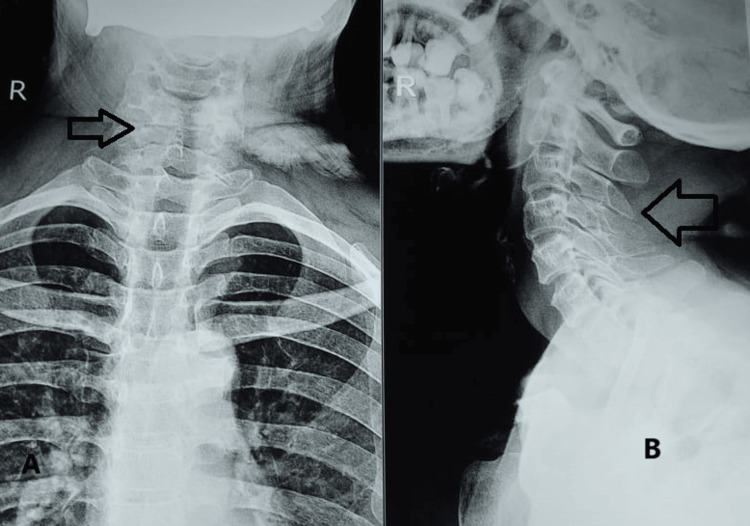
X-ray of the cervical spine in the anteroposterior (A) and lateral views (B) Evidence of a decreased gap between C6 and C7 as well as signs of wear and tear were observed in both A and B as shown by the arrows.
